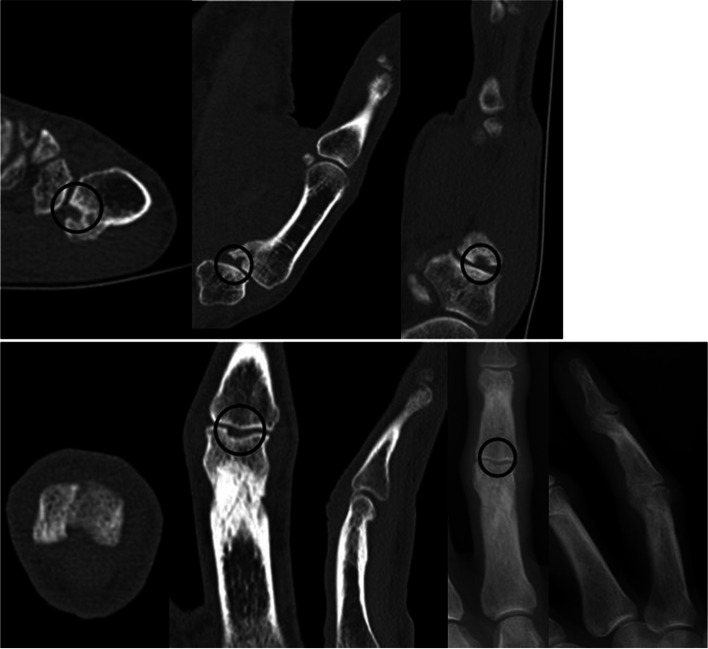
Top: Preoperative CT (left) and conventional radiographs (right) of malunited intra-articular fracture of the proximal interphalangeal III joint; bottom: Preoperative CT of malunited intra-articular fracture of the proximal metacarpophalangeal I joint
